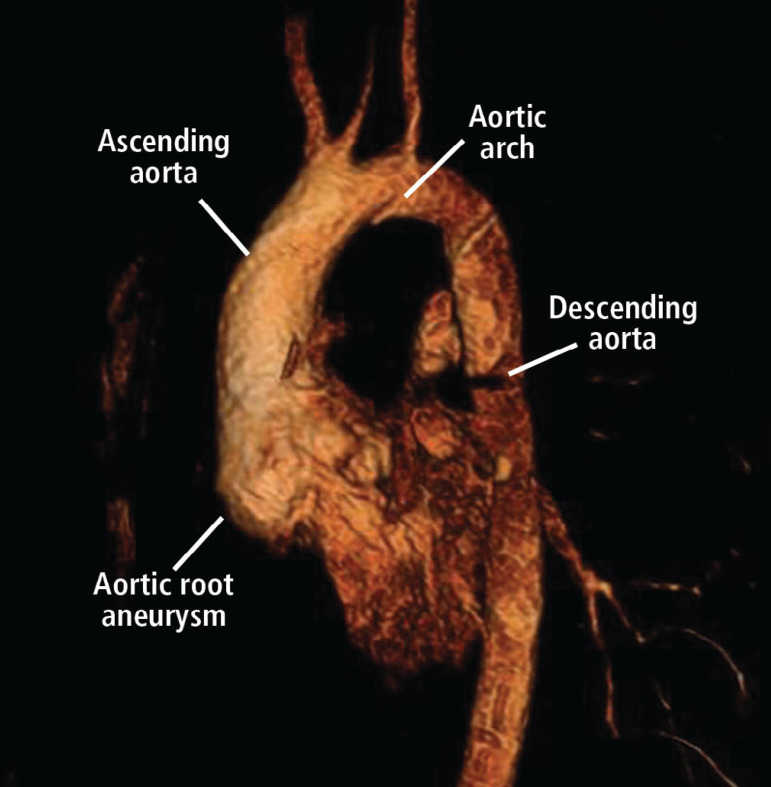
A three-dimensional computed tomography of aorta showing aortic root aneurysm in a patient with Marfan syndrome^[13]^.
